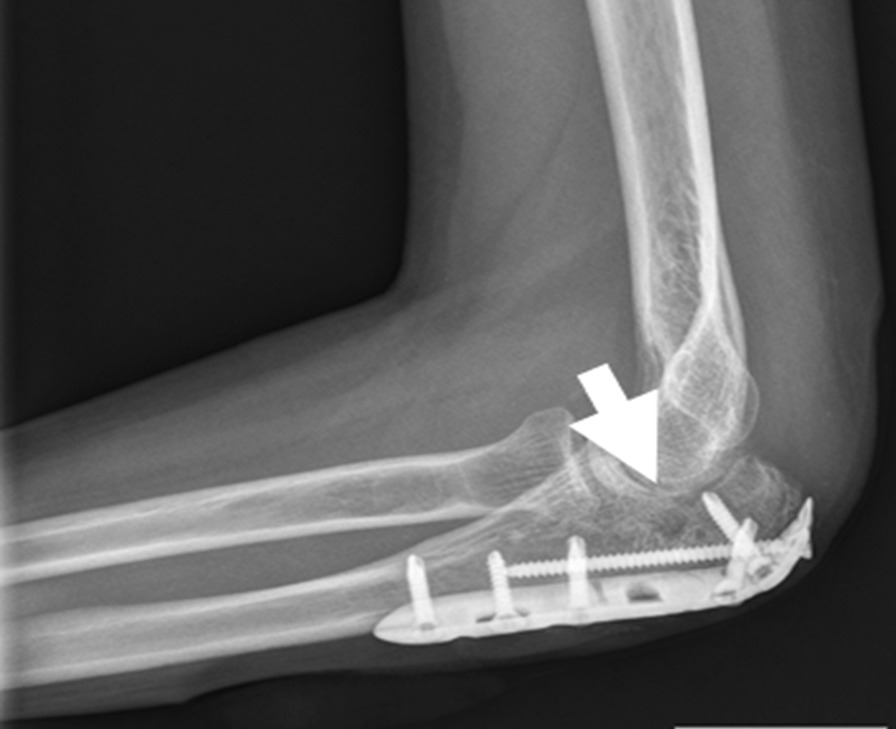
The X-ray reveals a shrinkage of the nidus-like lesion (white arrow) accompanied with initial bone callus formation
